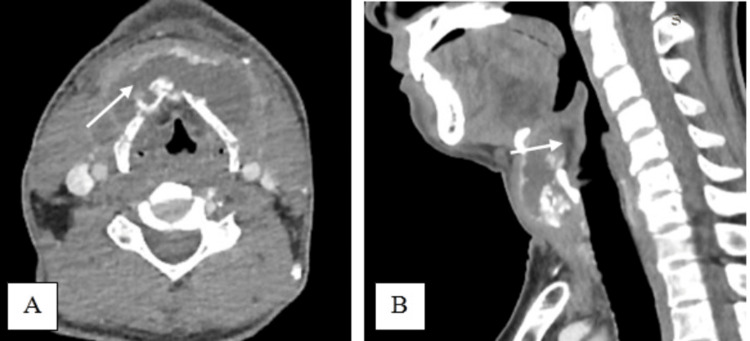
Axial view: Contrasted CT scan of the neck showing density fluid collection arising from perichondrium anterior to thyroid lamina with amorphous and multilobulated course calcification seen with erosion bilateral thyroid cartilage (white arrow) (A) Sagittal view: Contrasted CT scan of the neck showing collection extended into pre-epiglottic space (white arrow) (B).
